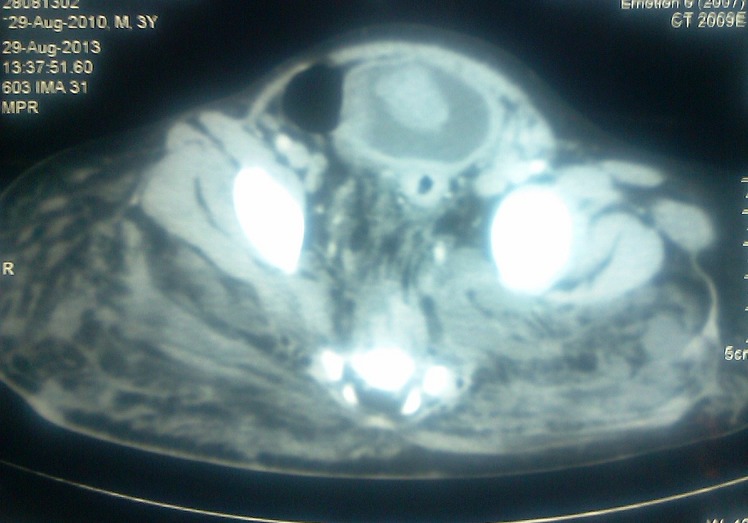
Figure 1: CT scan image shows soft tissue mass arising from dome of the urinary bladder.
